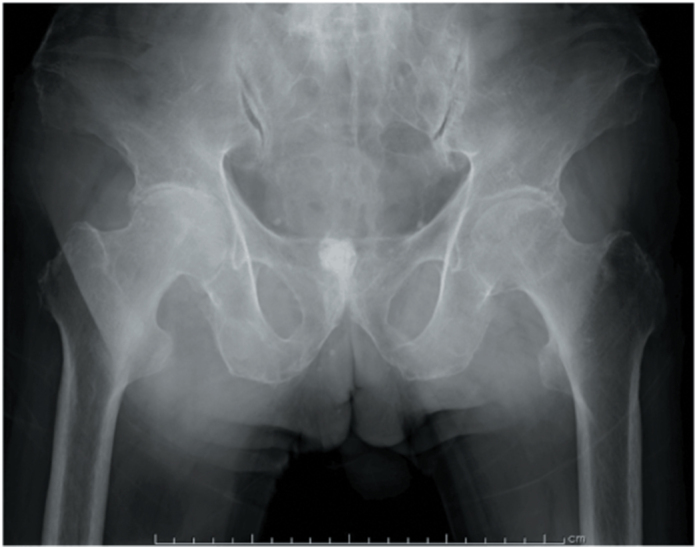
An anteroposterior radiograph of the hip joint at the first visit. A slight narrowing is
